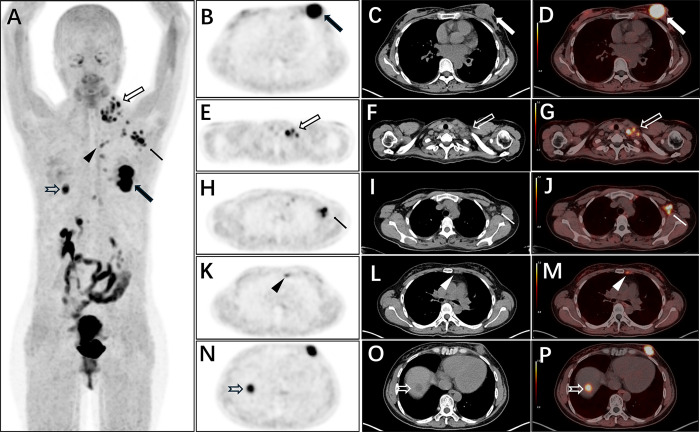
Al¹⁸F-NOTA-FAPI-04 PET/CT images of a 38-year-old female patient with breast invasive ductal carcinoma (molecular subtype: LuminalB). (A) Whole-body maximum-intensity projection (MIP) image shows multiple FAPI-avid lesions. Solid thick arrows in axial PET (B), CT (C), and fused PET/CT (D) images indicate the primary breast tumor. Open thick arrows in axial PET (E), CT (F), and fused PET/CT (G) images denote supraclavicular lymph node metastases. Thin arrows in axial PET (H), CT (I), and fused PET/CT (J) images indicate axillary lymph node metastases. Triangles in axial PET (K), CT (L), and fused PET/CT (M) images indicate an internal mammary lymph node. Open arrowheads in axial PET (N), CT (O), and fused PET/CT (P) images indicate a hepatic metastasis.
